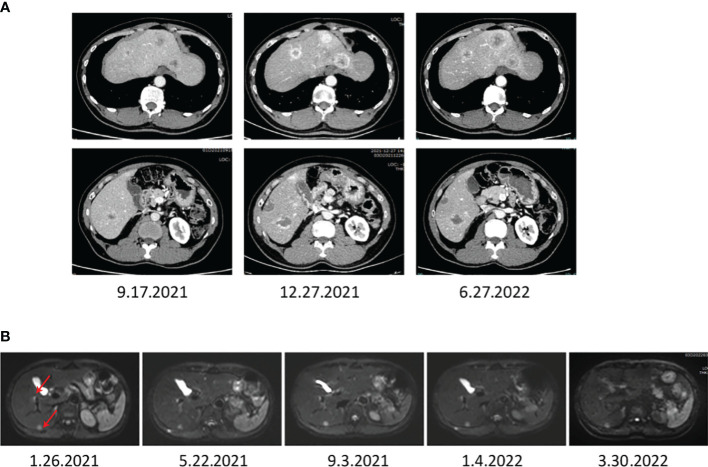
The images display the abdominal CT (A) and MRI (B) after a combination treatment of TACE, anti-PD-1 antibodies, and apatinib, showing that the liver metastasis of NETs diminished significantly.
